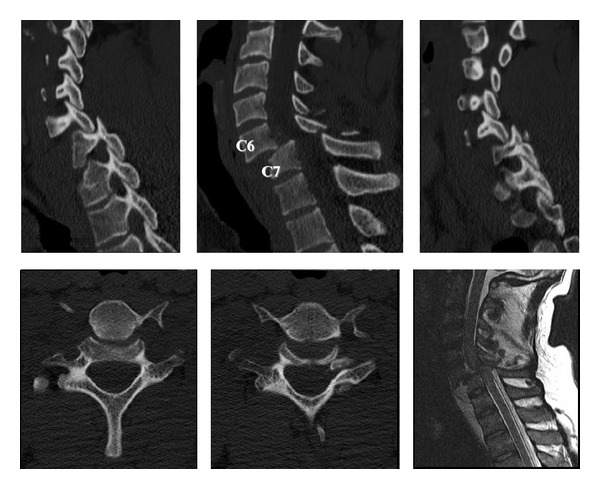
CT cervical scan showing a severe retrolisthesis of C6 on C5, with facet joint dislocation. MRI confirming the listhesis and demonstrating severe spinal cord injury with signs of myelopathy.
